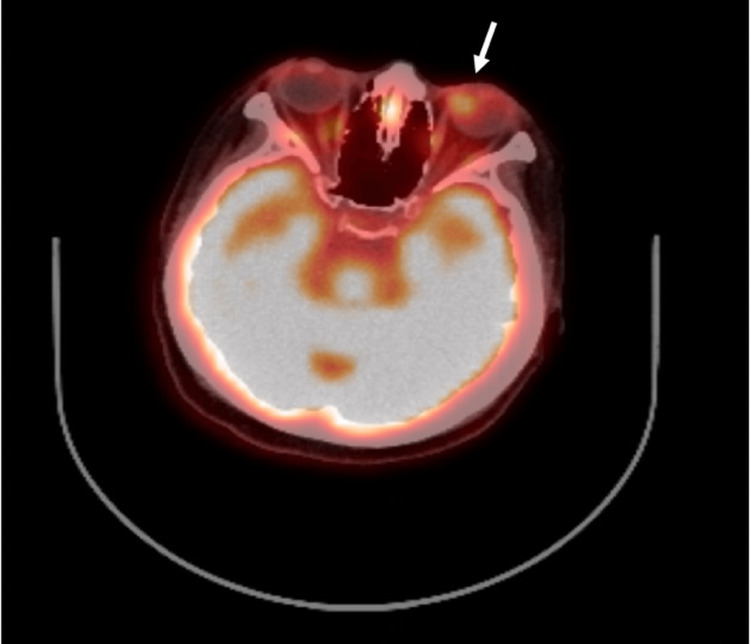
FDG-PET showing increased uptake in the left choroidal lesion (white arrow) and in the nasal septum.
